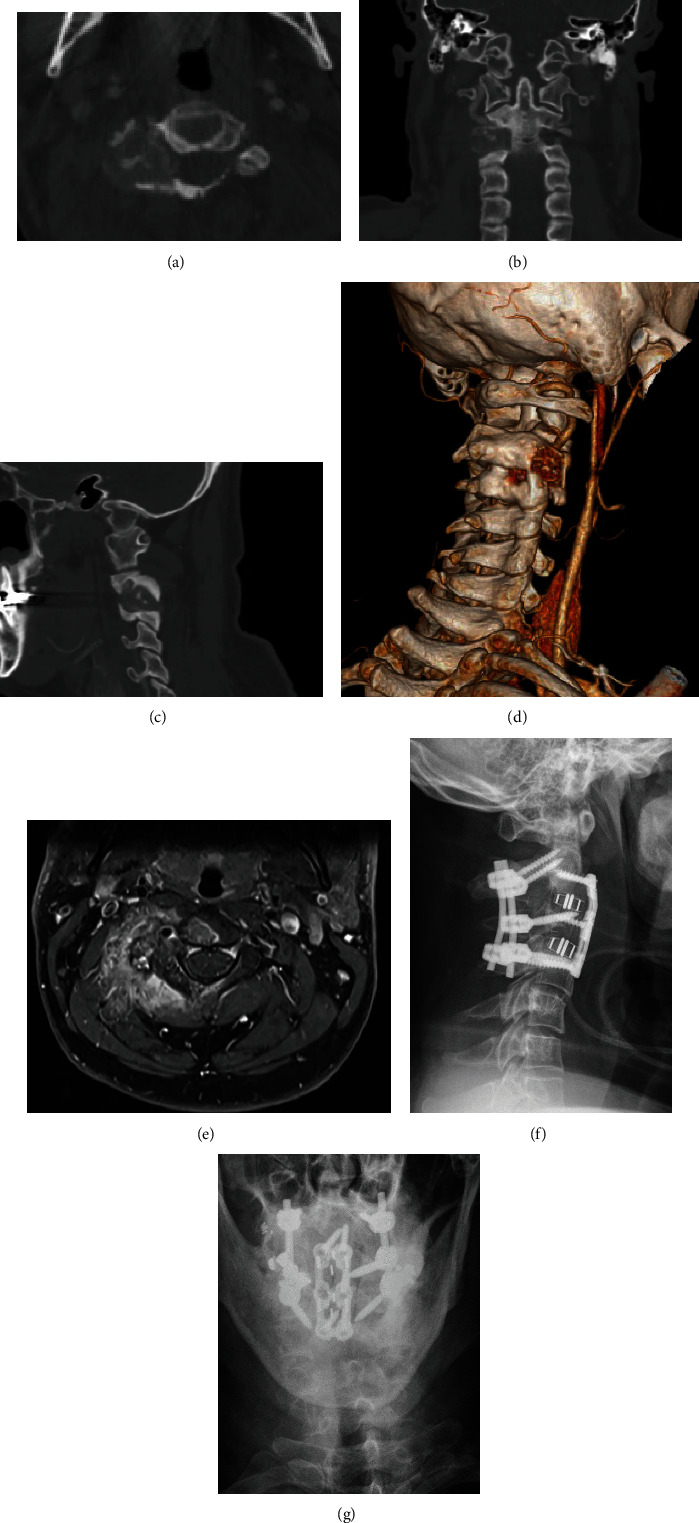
Case 2: aggressive osteoblastoma in C3. (a–d) CT scan reconstructions of the cervical spine with a right-sided lesion in C3. (e) MRI shows the soft-tissue component of the tumor. (f, g) Postoperative a.p. and lateral radiographs after resection of the tumor and combined posteroanterior stabilization.
